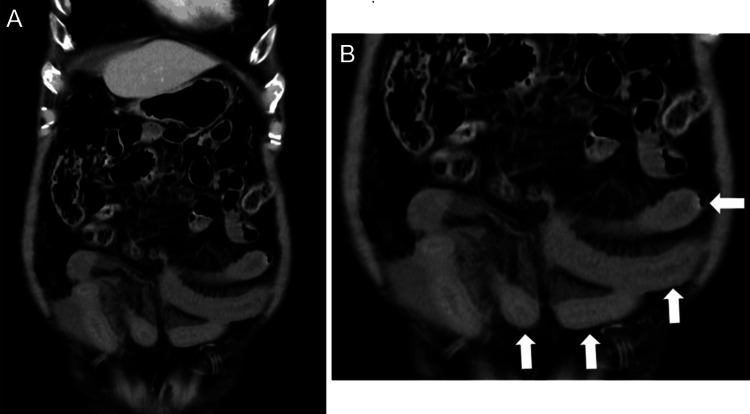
Extensive small intestinal ischemia suspected on contrast-enhanced CT (A) Contrast-enhanced abdominal CT scan. (B) Magnitude image showing dilated and edematous segments of the small intestine with poor enhancement (arrows), suggestive of strangulated small bowel obstruction, without evidence of lymph node enlargement.
